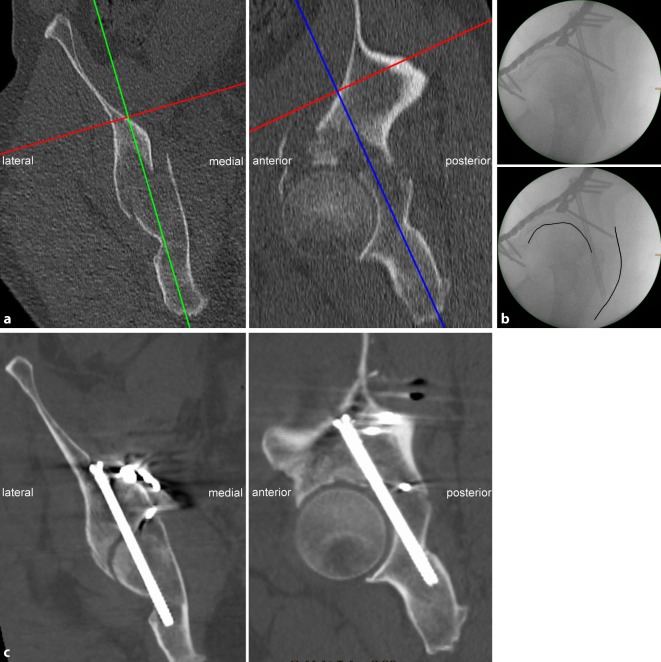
Screw misplacement. a Preoperative planning for a 56-year-old man who sustained a typical anterior column with posterior hemitransverse fracture (ACPHF) on the right side. The preoperative planning reveals a relatively narrow osseous corridor for a posterior column screw. b Fluoroscopic control in lateral–oblique view. The image quality is moderate in general. Additionally, the lateral–oblique view was not sufficiently oblique resulting in a poor differentiation between the left and the right hip and sciatic tuber (*upper image*). The screw does not perforate the cortical bone at the sciatic tuber, but is tangent to the projection of the hip joint line (*lower image*). c Postoperative CT scans revealed adequate reduction of the posterior hemitransverse fracture via the quadrilateral plate. The posterior column screw, however, penetrates the cortex of the acetabular fossa. The hip joint motion was not restricted and the patient refused revision surgery
